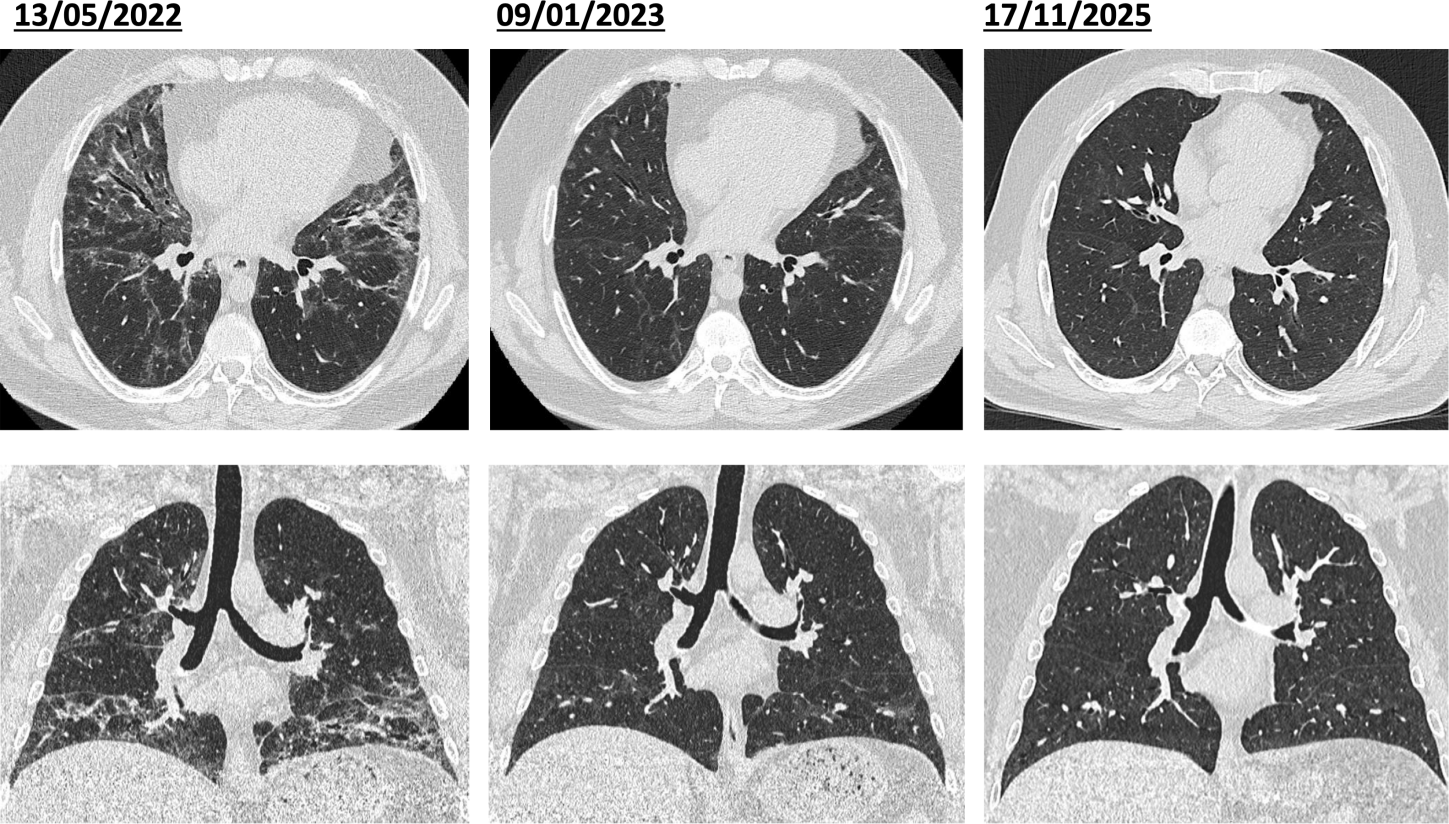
Relapse and response to rituximab*. Left: Recurrence despite maintenance therapy with Azathioprine with similar changes to the initial CT characterized by extensive reticulation and interstitial thickening, along with ground-glass opacities and bronchiectasis in both lungs, predominantly affecting the basal regions. Middle: Near-total resolution of the ground-glass opacities and bronchiectasis after escalation of therapy to Rituximab. Right: morphological normalization of the lung parenchyma under maintenance monotherapy with Rituximab.
